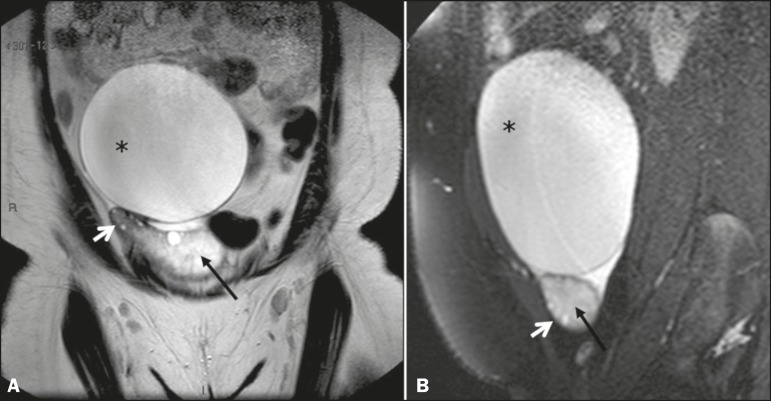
Coronal and sagittal T2- weighted MRI sequences (A and B, respectively). A: Large adnexal cystic formation (paraovarian cyst, asterisk). Note the hyperintense area, consistent with stromal edema, in the center of the ovary (black arrow), with consequent displacement of follicles to the periphery (white arrow). B: Another large adnexal cystic formation (paraovarian cyst, asterisk) . Again, there is a hyperintense area, consistent with stromal edema, in the center of the ovary (black arrow), with consequent displacement of follicles to the periphery (white arrow).
